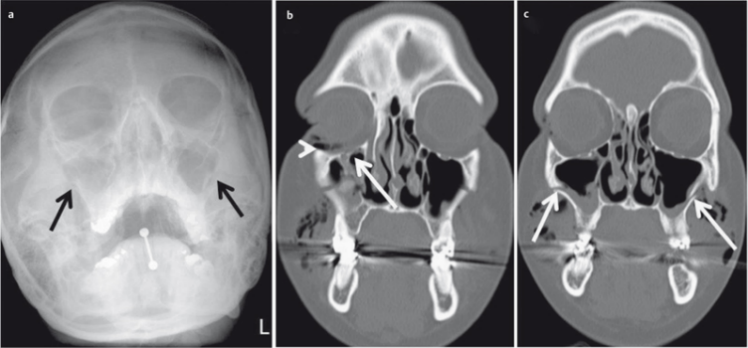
22-year-old drunken patient having received blows to the face. a) Conventional radiography of the paranasal sinuses. Suboptimal image quality due to lack of compliance. There is a fracture of the lateral aspects of both maxillary sinuses (arrow), additional fractures are not detectable. b) CT of the midface, coronal plane; fracture of the floor of the orbit (arrow) and intraorbital air (arrowhead). c) CT of the midface allows precise determination of the extent of the fracture (arrow).
